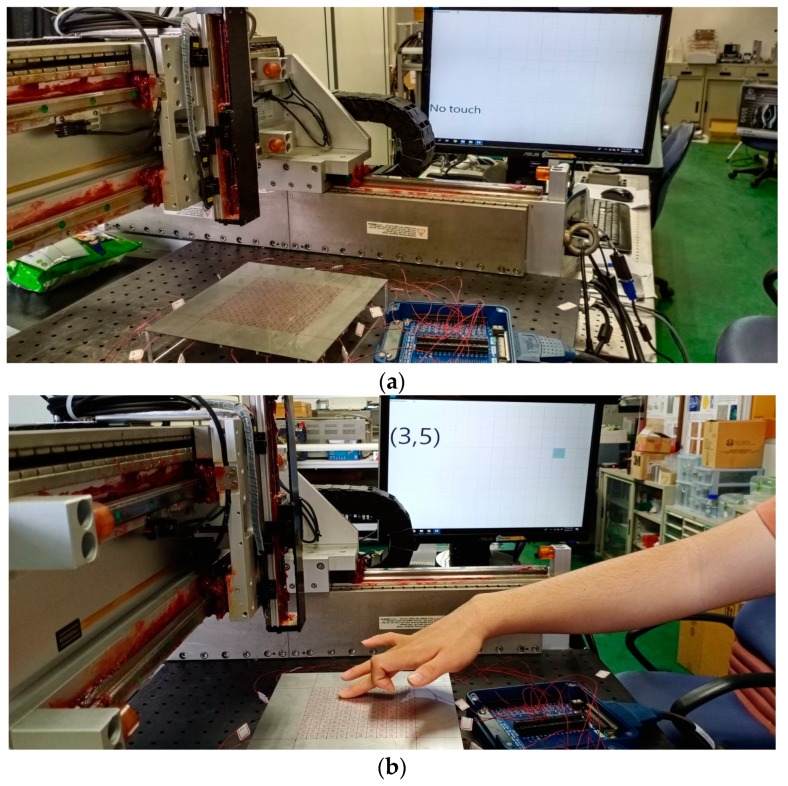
Photos of human-finger testing on the Lamb-wave ultrasound touchscreen when there is (a) no touch and (b) finger touch at the position coordinates of (3, 5) on the steel plate.
